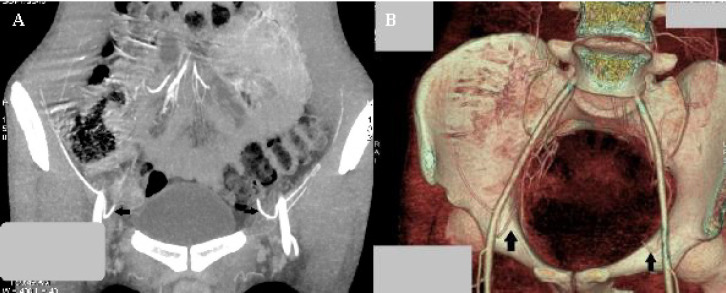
CT angiographic images in a 68-year-old man showing the bilateral Corona Mortis arteries (arrow). (A) Coronal MIP image (B) oblique 3D volume rendered image
